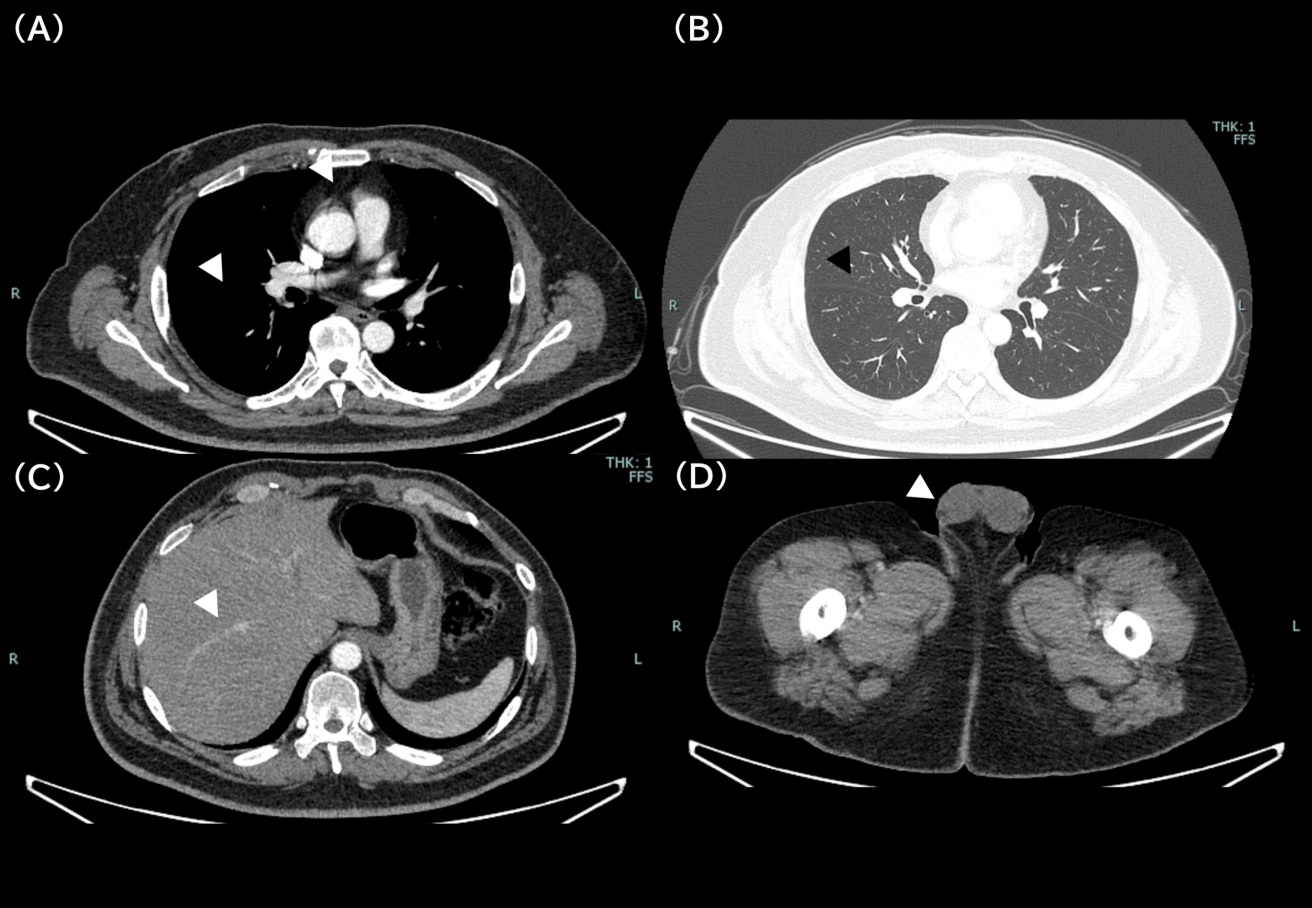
Whole-body computed tomography (CT) findings. Contrast-enhanced CT scans covering the chest (A, B), abdomen (C), and pelvis (D). The arrowheads indicate the normal-appearing lung fields, thymus, liver, and testes. These annotations are provided to explicitly demonstrate the absence of occult mass lesions in organs frequently associated with paraneoplastic neurological syndromes, such as thymoma or testicular germ cell tumors.
